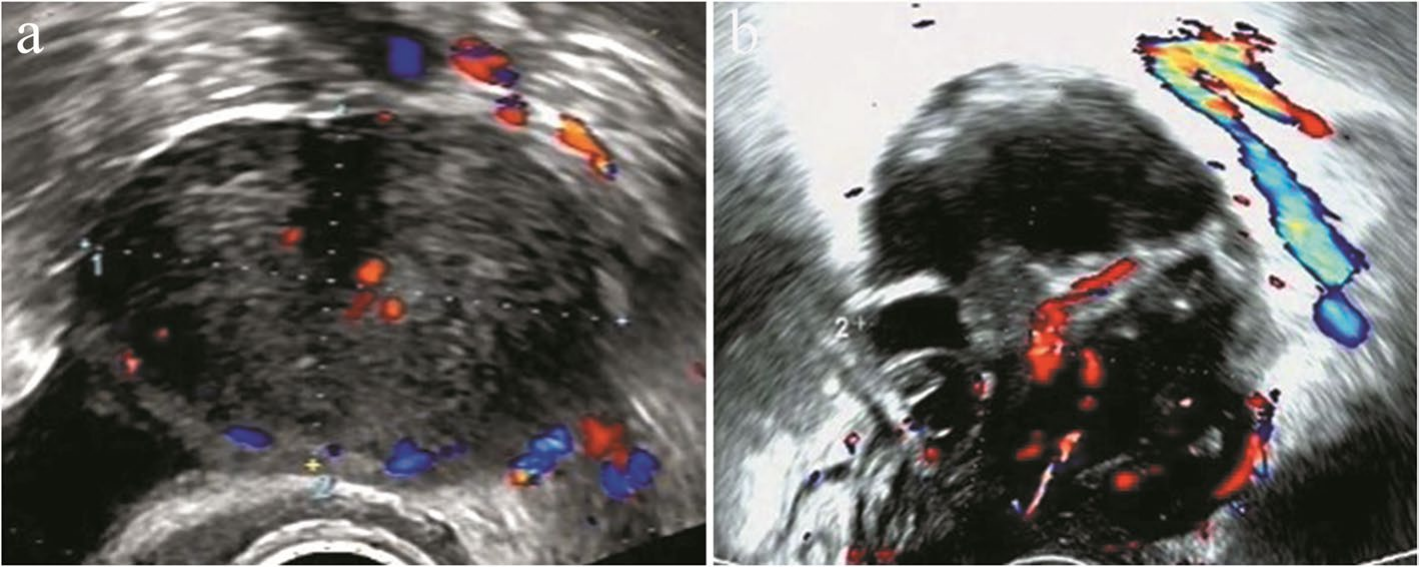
Ultrasound images supporting the difference in results between the groups. a. Image of a pathologically proven adult granulosa cell tumor from a patient (case 172 among the 599 women) is shown. This was a smooth solid mass with a color score of 2–3. Both senior and junior doctors classified the mass as O-RADS 4. But Senior doctors subjectively diagnosed it as malignant, while junior doctors subjectively diagnosed it as benign. b. Image of a pathologically proven mucinous cystadenoma from a patient (case 204 among the 599 women) is shown. This was a multilocular cyst with solid component, and with a color score of 3–4. Senior doctors classified the mass as O-RADS 5, and junior doctors classified it as O-RADS 4. Both senior and junior doctors diagnosed the mass as malignant by subjective judgment
